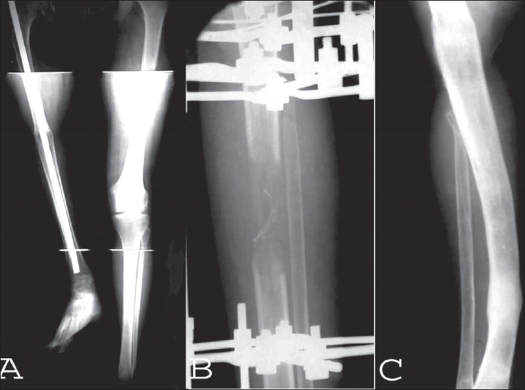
A) Combined x-ray of a patient who underwent segmental resection of limb with telescoping on an intramedullary nail. Note the marked residual shortening. B) First stage tibial lengthening being performed after nail removal. C) Final outcome of the patient after second stage lengthening
